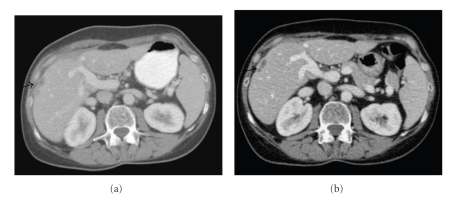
Postoperative CT-scan showing early disease recurrence (indicated with an arrow) within the liver (Figure 2(a)). Partial response after completion of chemotherapy is shown in Figure 2(b).
